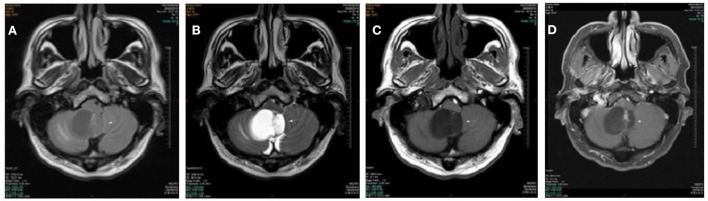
Cases 5: cerebellar hemangioblastomas. Axial T1WI (A), axial T2WI (B), and axial FLAIR (C) show a cyst mass in the cerebellar hemisphere and vermis with the marked hypointense signal on T1WI, the hyperintense signal on axial T2WI, and iso- and hypointense on FLAIR. The lesion shows septum and nodule enhancement on postcontrast axial T1WI with fat saturation (D).
